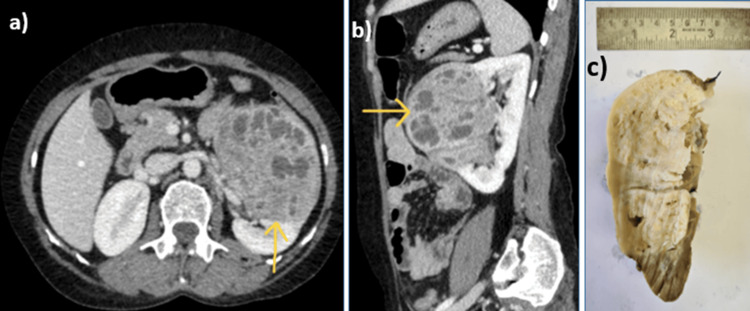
Axial and sagittal CT (a, b) and gross findings of the left kidney (c) Axial and sagittal CT views showing a lesion in the interpolar and upper pole of the kidney (a, b). Gross findings of the left kidney showing multiple cystic and solid areas in the interpolar and upper pole of the kidney (c).
